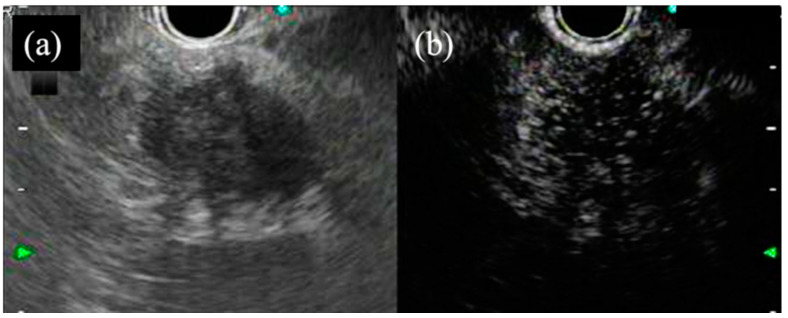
A typical example of pancreatic adenocarcinoma with hypoenhancement. Fundamental B-mode endoscopic ultrasound (EUS) (a) showing a hypoechoic tumor at the pancreatic body, and contrast-harmonic EUS (b) showing the tumor with hypoenhancement.
